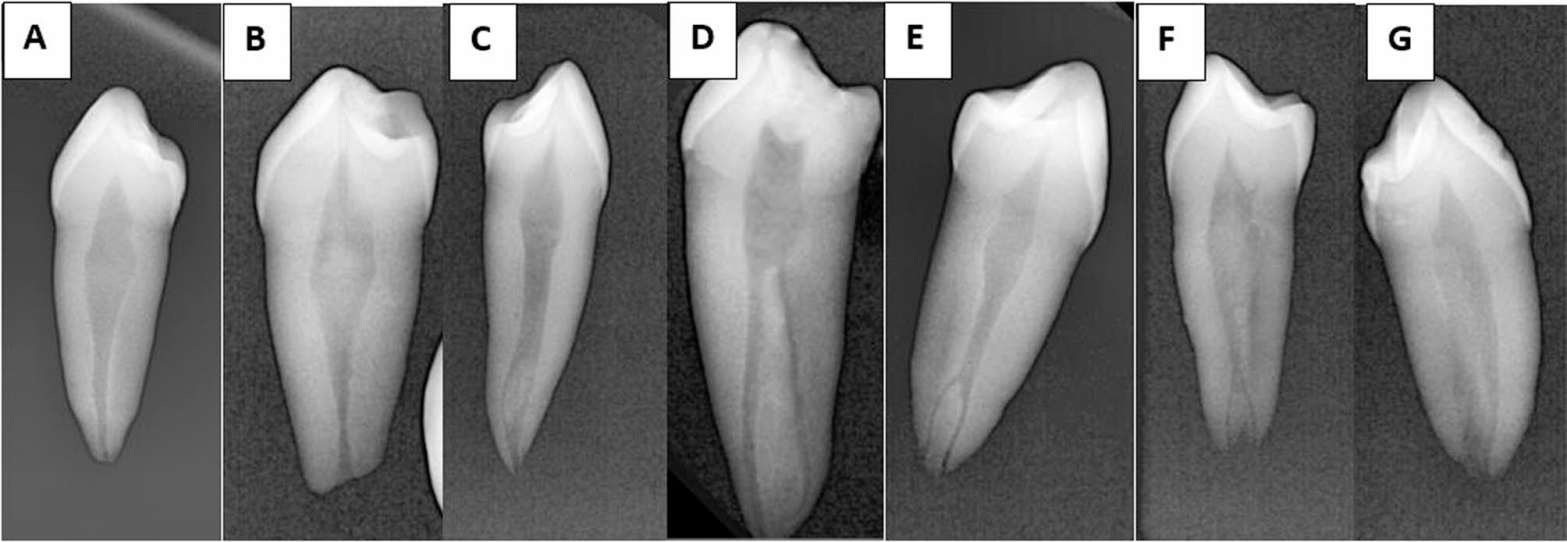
Examples of digital periapical radiographs of mandibular premolars: A: with Vertucci Type 1, B: with Vertucci Type II, C: with Vertucci Type III, D: with Vertucci Type IV, E: with Vertucci Type V, F: with Vertucci Type VI, and G: with ^2^TN^1^B^1^L^1^ according to Ahmed Classification
